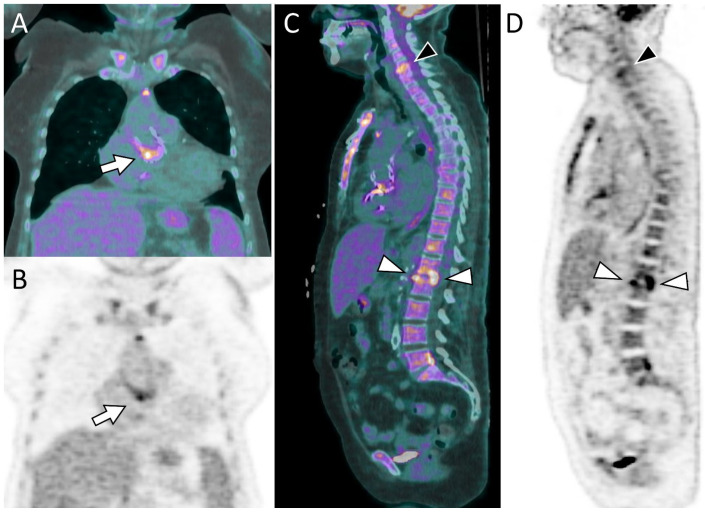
A 76-year-old woman with a Perceval prosthetic aortic valve and positive blood cultures for *Enterococcus faecalis*. Echocardiography revealed a small mobile structure that was inconclusive for vegetation, prompting further evaluation with [^18^F]FDG PET/CT. Fused (A) and attenuation-corrected (B) coronal images demonstrate focal and intense FDG uptake at the level of the Perceval prosthesis (arrows), consistent with prosthetic valve endocarditis. (C,D) Whole-body imaging additionally revealed findings consistent with spondylodiscitis at the L1–L2 (white arrowheads) and C5–C6 (black arrowhead) levels.
